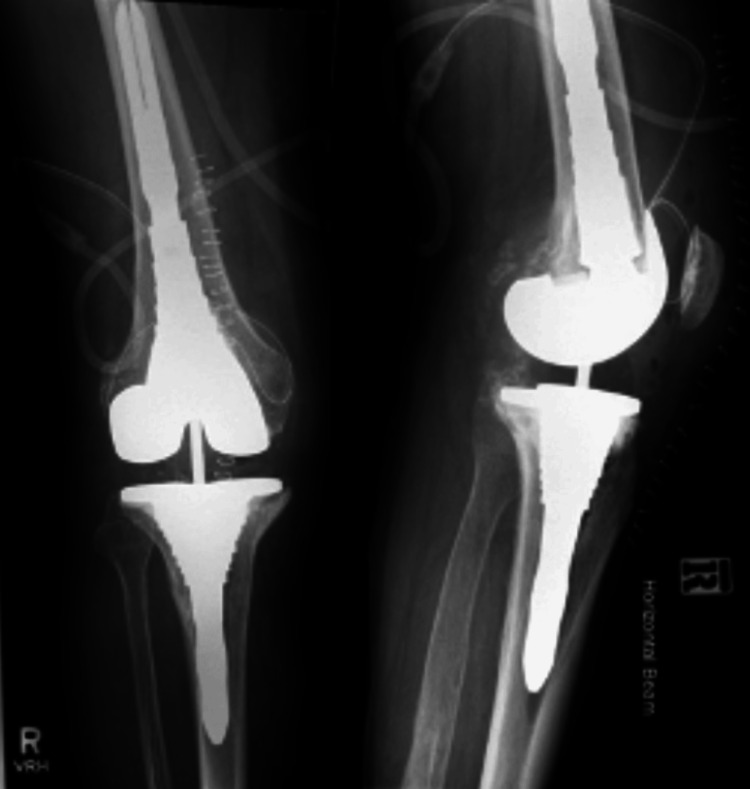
Postoperative anteroposterior and lateral X-rays.
